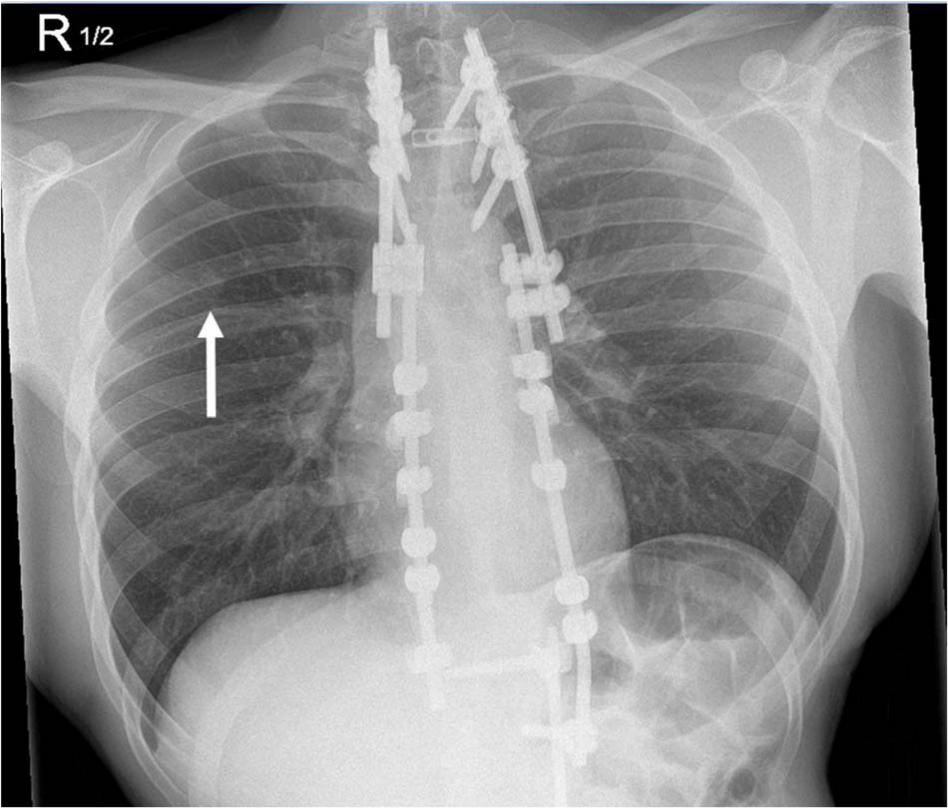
A monitoring radiograph 46 months after initial surgery noted a new nine-millimetre nodule in the right upper lung.
